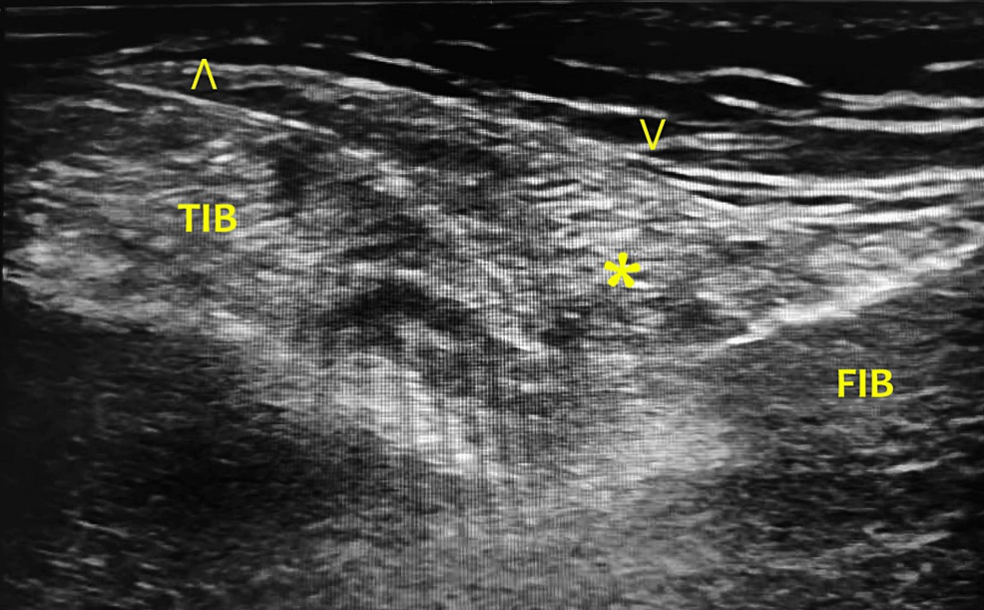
Sonographic view of in-plane PTFJ injection ˄ signifies the needle in plane, ˅ signifies the PTFJ capsule, and * signifies the injectable inside the PTFJ. FIB, fibula; TIB, tibia
